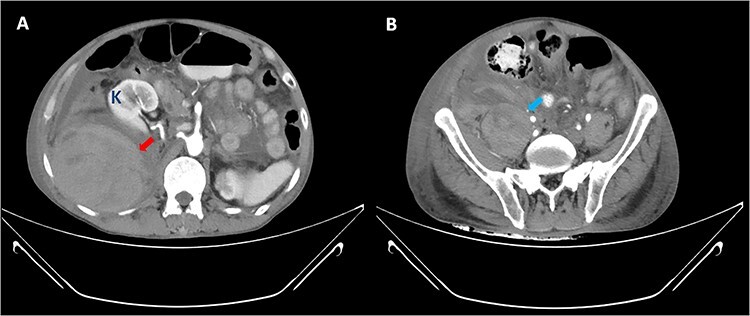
CT abdomen axial view shows large right retroperitoneal hematoma (red arrow) and right psoas hematoma (blue arrow) with displacement of right kidney (K) anteriorly.
